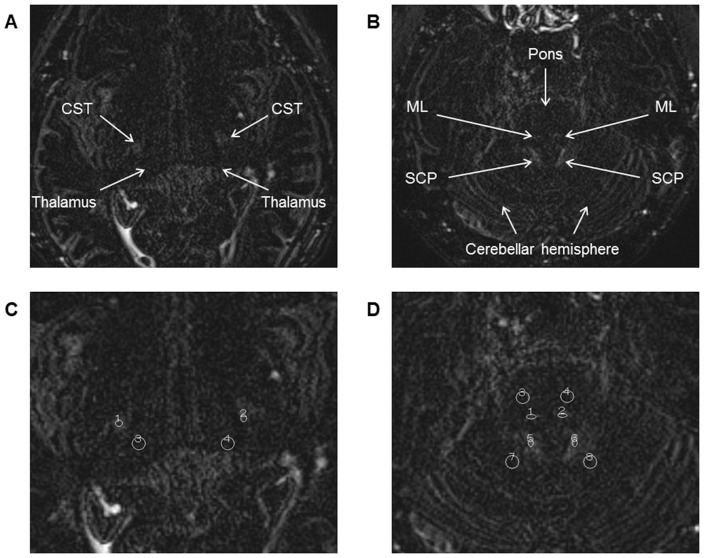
The regions of interest setting on delayed enhanced-heavily T2-weighted three-dimensional fluid-attenuated inversion recovery (DE-hT2w-3D-FLAIR) images. Axial, delayed enhanced, heavily T2-weighted three-dimensional fluid-attenuated inversion recovery (hT2w-3D-FLAIR) images from the same patient as in Figure 2. Images were obtained 4 h after intravenous gadolinium injection. Images on the left side (A and C) are at the levels of the thalamus (Th), and images on the right side (B and D) are at the level of the trigeminal nerve. The corticospinal tract (CST), medial lemniscus (ML), and superior cerebellar peduncle (SCP) are visualized as areas of high signal intensity (A and B). Images C and D are trimmed magnifications of images A and B, with regions of interest (ROI) setting. Oval 5-mm^2^ ROI were set on the CST, ML, SCP, and circular 15-mm^2^ ROI were set on the Th, pontine parenchyma (PP), and cerebellar parenchyma (CP) (C and D). To evaluate the signal contrast between white matter (WM)-tracts and contiguous brain parenchyma, the following signal intensity ratios between WM-tracts and contiguous brain parenchyma were calculated: the ratio of the signal intensity of the CST to the signal intensity of the Th on the same side (CThR), the ratio of the signal intensity of the ML to the signal intensity of the PP on the same side (MPPR), and the ratio of the signal intensity of the SCP to the signal intensity of the CP on the same side (SCPR).
